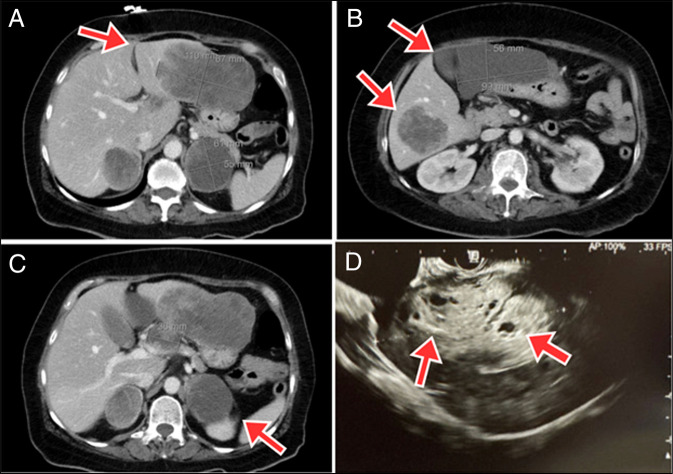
(A–C) Axial contrast-enhanced computed tomography images of the abdomen showing a large exophytic heterogeneous mass at the pancreatic head (3.7 × 3.2 cm) abutting the caudate lobe of the liver. Extensive metastatic disease is noted, including large lesions in the liver, bilateral adrenal glands, and retroperitoneum (red arrows). (D) EUS of the liver demonstrating multiple hypodense lesions (red arrows) consistent with hepatic metastases. Notably, no pancreatic mass or pancreatic ductal dilation was visualized on EUS. EUS, endoscopic ultrasound.
